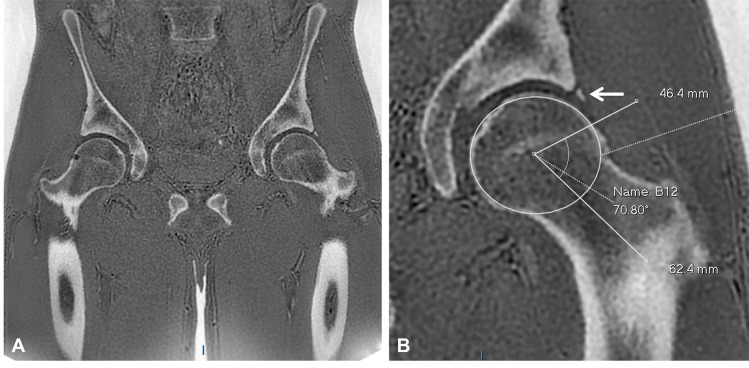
Coronal ZTE MRI of the pelvis and hips for assessment of osseous morphology in an athlete. (A) The coronal ZTE image demonstrates bilateral hip osseous anatomy with improved cortical definition. (B) Magnified view of the left hip demonstrates an osseous bump at the femoral head–neck junction in keeping with CAM morphology, an increased alpha angle of 71°, and an os acetabuli (arrow).
